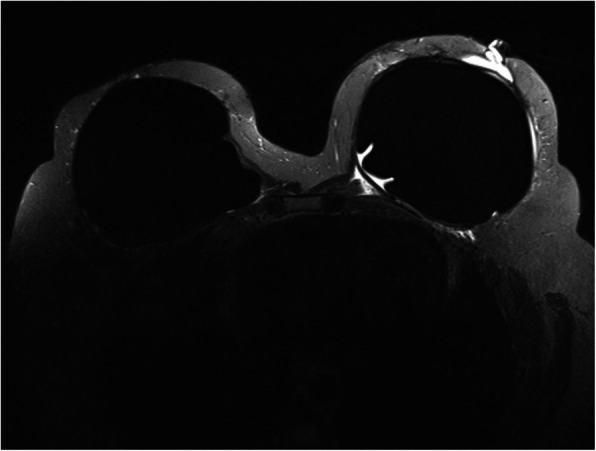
Axial T2-weighted image of the left breast with fat suppression after contrast obtained showing a recurrent fluid collection extending into the nipple ducts
